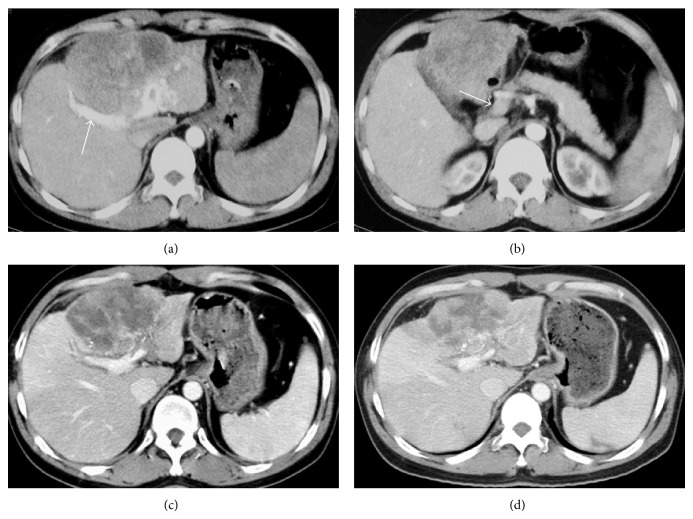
CT images. (a) and (b) refer to images at the initial presentation. (a) Shows a hypoattenuating mass with areas of heterogeneous enhancement in the left hepatic lobe. Visible right branch of portal vein was present in arterial phase axial CT image ((a), arrow), whereas main portal vein showed little contrast enhancement on arterial phase imaging ((b), arrow). (c) and (d) demonstrate the decreases of tumor diameter after the treatment at 59 and 152 days after the diagnosis, respectively.
